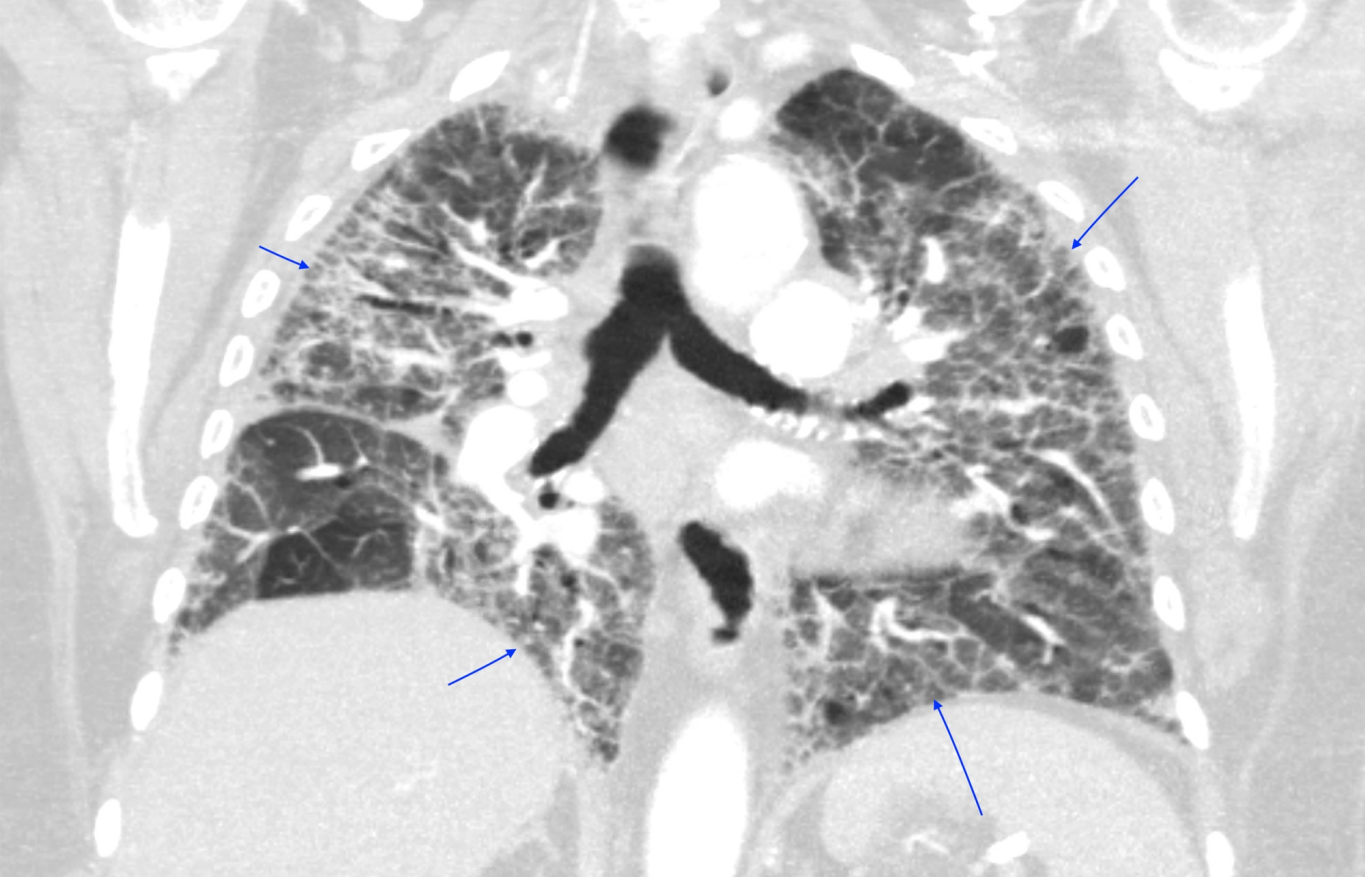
CT Chest (Coronal View) Progression of bilateral alveolar opacities, interlobular septal thickening, and ground-glass attenuation throughout the lungs without intraluminal defects.
